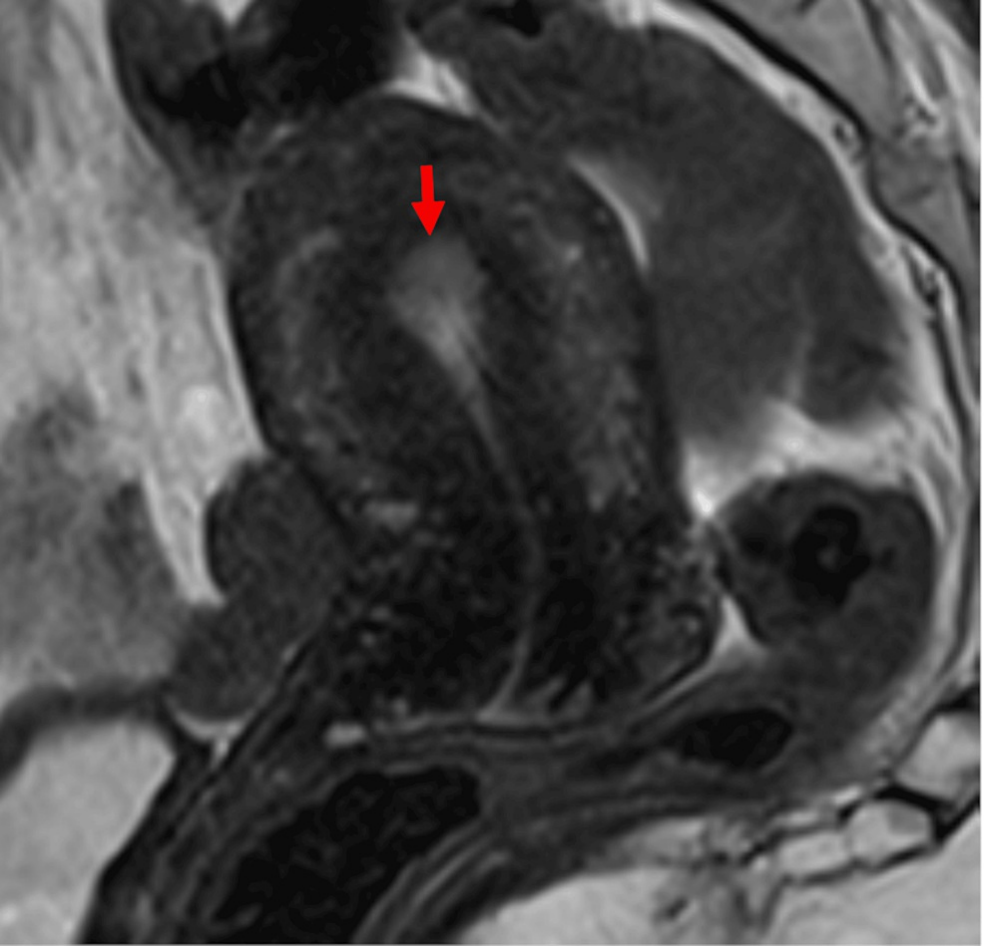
Midsagittal section of the uterus T2-weighted imaging on MRI shows an 8 x 13 mm endometrial tumor (red arrow) without myometrial invasion and indicates stage IA endometrial cancer. MRI: magnetic resonance imaging
